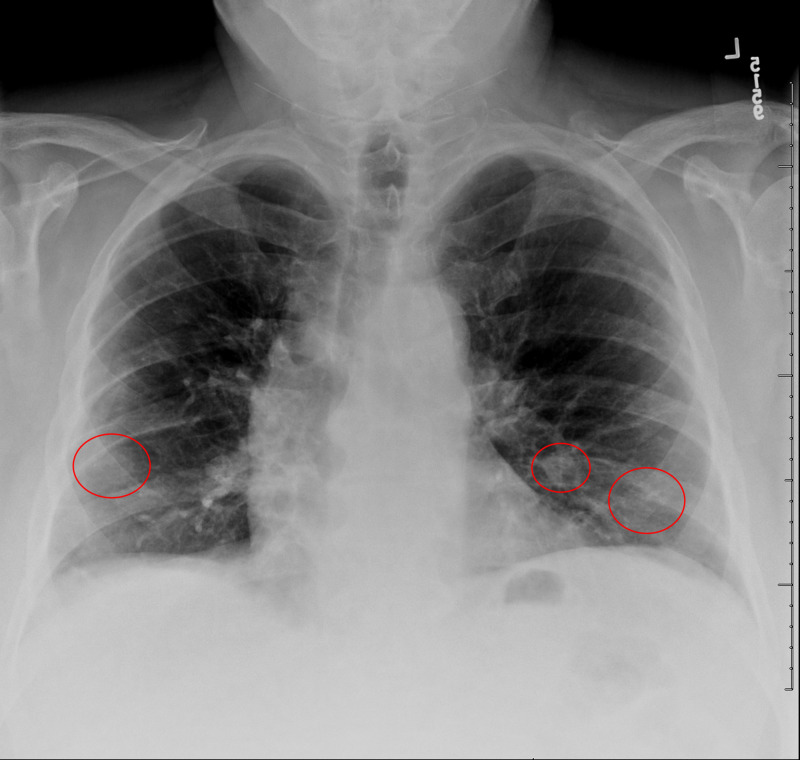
Chest X-ray of the patient Subtle hazy airspace opacities (red circles) in bilateral lower lungs suspicious for infections including COVID-19 pneumonia COVID-19: coronavirus disease 2019
